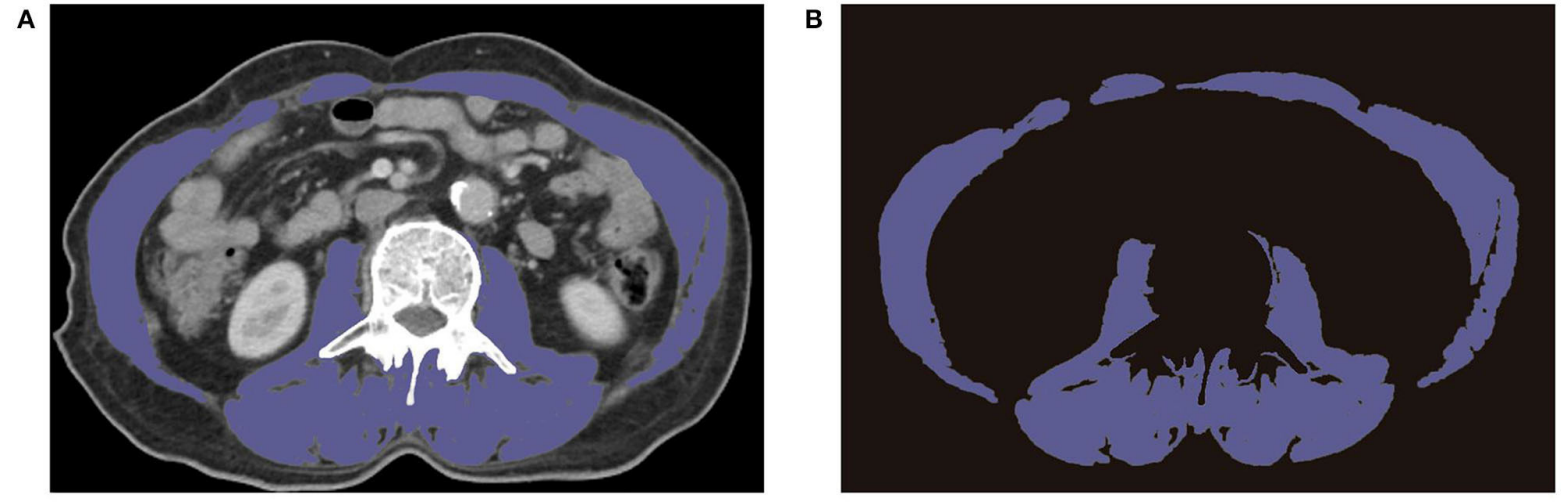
Preoperative CT images of L3 region showing the border of skeletal muscle area. (A) Representative CT images on the level of L3. (B) Extracted images of skeletal muscle on the level of L3.
